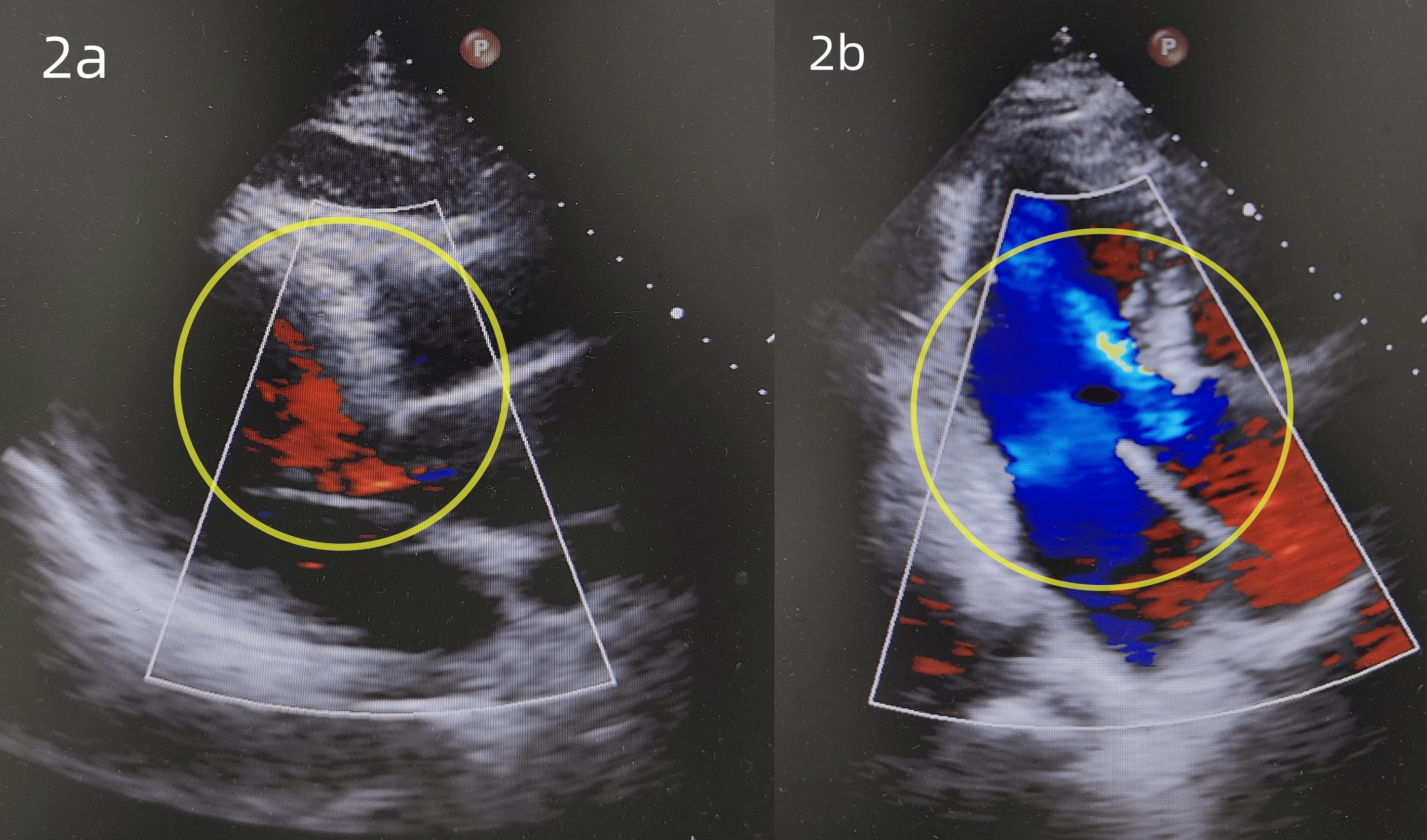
Cardiac ultrasound. (a) The yellow circle indicates left heart chamber enlargement. (b) The yellow circle indicates aortic dilation.
